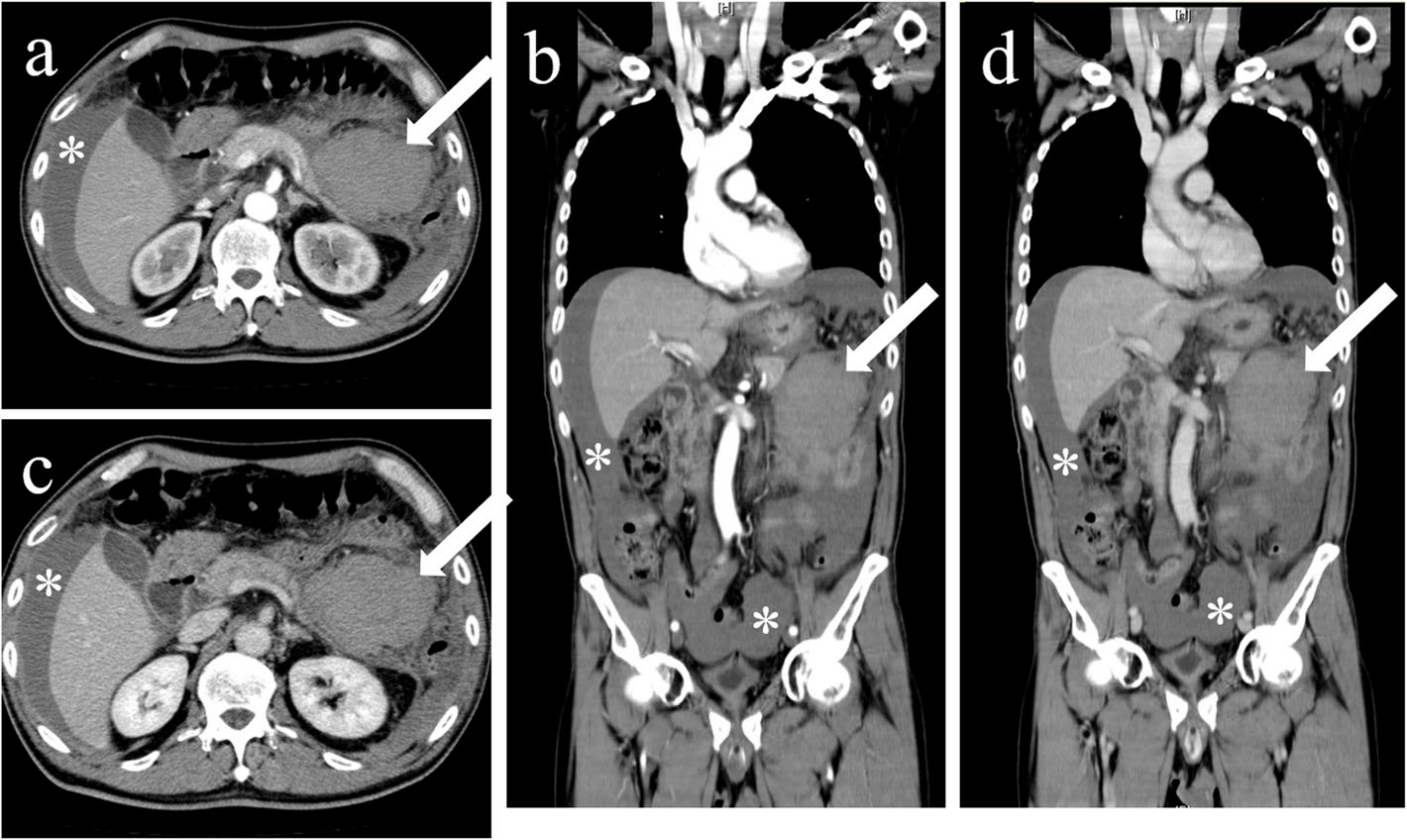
A computed tomography with contrast on arrival at our emergency department is shown; a an axial section and b a coronal section in the early phase, and c an axial section and d a coronal section in the late phase. A non-enhanced, homogenous, 8.5 cm in diameter, high-density mass lesion is located between the posterior wall of the stomach and the anterior side of the pancreatic tail (arrows). There was no sign of extravasation or aneurysmal rupture. A large amount of intraabdominal fluid collection is demonstrated (asterisks). Note that the amount of the fluid collection increased compared to the initial plain CT
